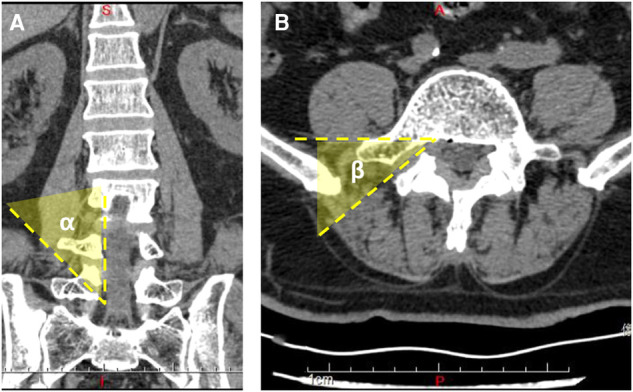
(A) Three-dimensional computed tomography scan shows the angle of trajectory in the coronal plane. (B) Three-dimensional computed tomography scan shows the angle of trajectory in the sagittal plane.
